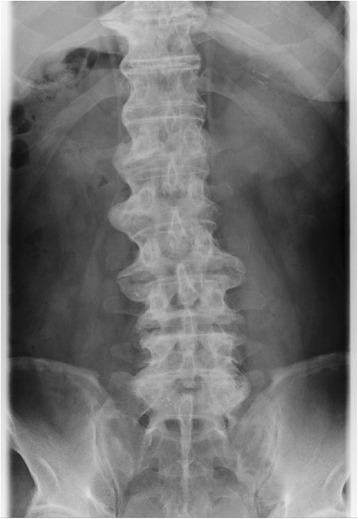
Plain radiographs of the lumbar spine. Antero-posterior view shows non-specific, mild to moderate degenerative changes
